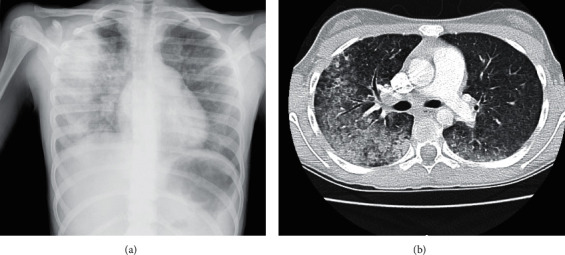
Chest X-ray (a) and CT scan (b) showing signs of alveolar hemorrhage, Case 2.
